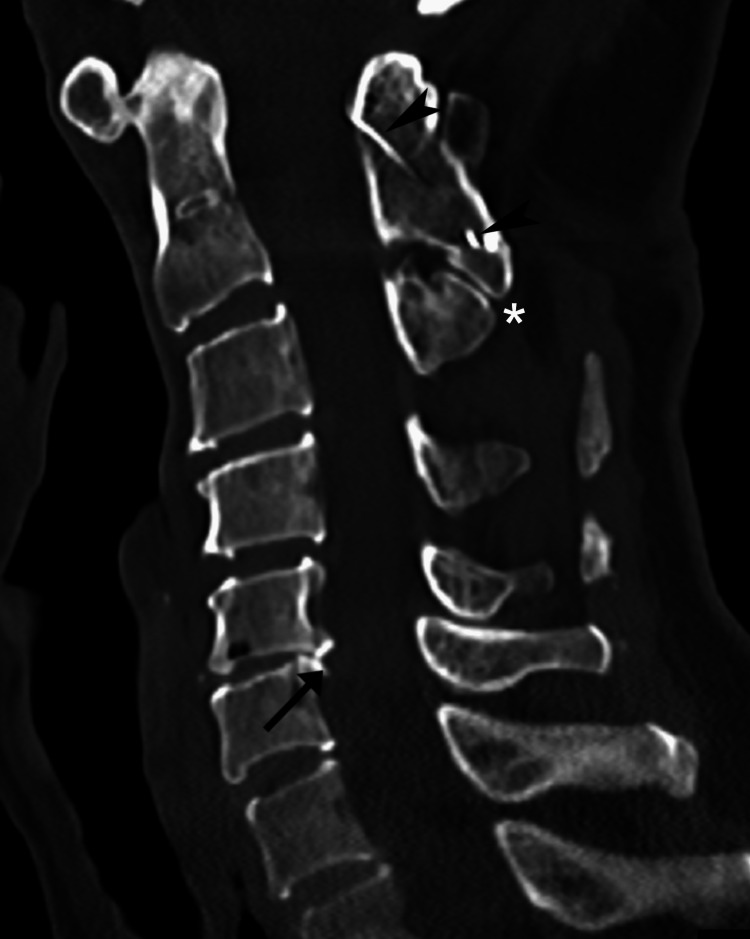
Cervical spine computed tomography, mid-sagittal section Cerclage wire (arrowheads) is evident from her previous procedure which was placed from the C1 posterior arch to the C2 spinous process with interposed autograft bone. Mild disc herniations are evident at C3/4 and C4/5, while the C5/6 disc space is severely narrowed and posteriorly has a disc-osteophyte complex (arrow), which causes mild narrowing of the spinal canal at this level to 8 millimeters in anteroposterior diameter and mild osteophytic encroachment of the left C5/6 neuroforamina (not shown). Interspinous narrowing and pseudoarticulation is evident between the C2 and C3 spinous processes (*). A slight focal kyphosis from C1 to C4 is also evident.
